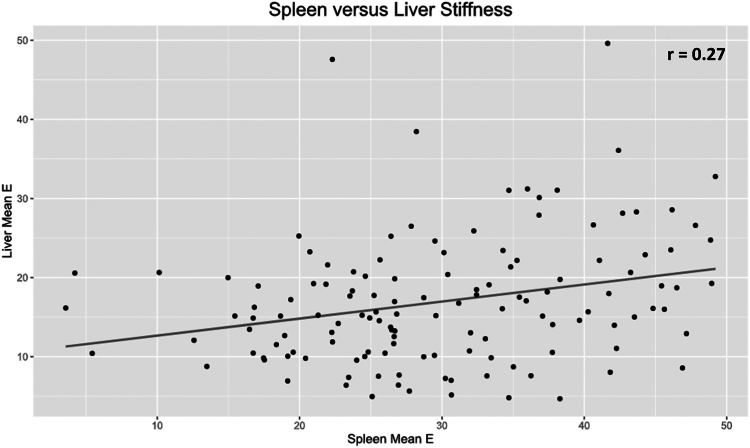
Correlation between mean spleen and liver stiffness measurements obtained using endosonographic shear wave elastography. *E*, Elastic modulus.
